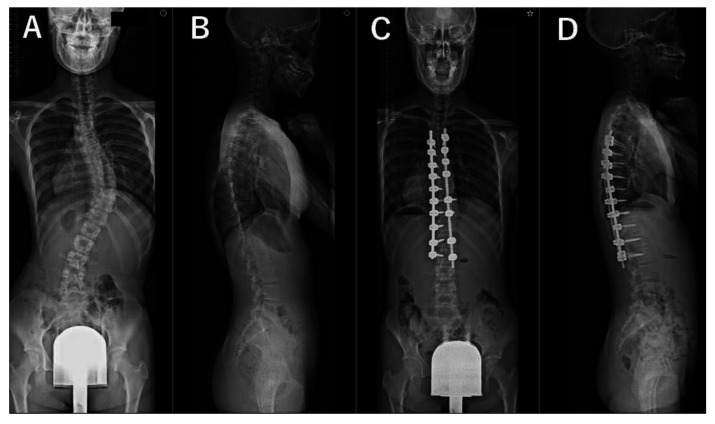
Pre- (A,B) and post- (C,D) operative radiographs of representative case with 12-year-old female patient. Cases of preoperative cervical lordosis changed to postoperative cervical lordosis. Cervical lordosis (1°, 23°), thoracic kyphosis (21°, 20°), lumbar lordosis (44°, 46°), T1 slope (13°, 19°), major thoracic curve (43°, 2°). (Preoperative, postoperative).
